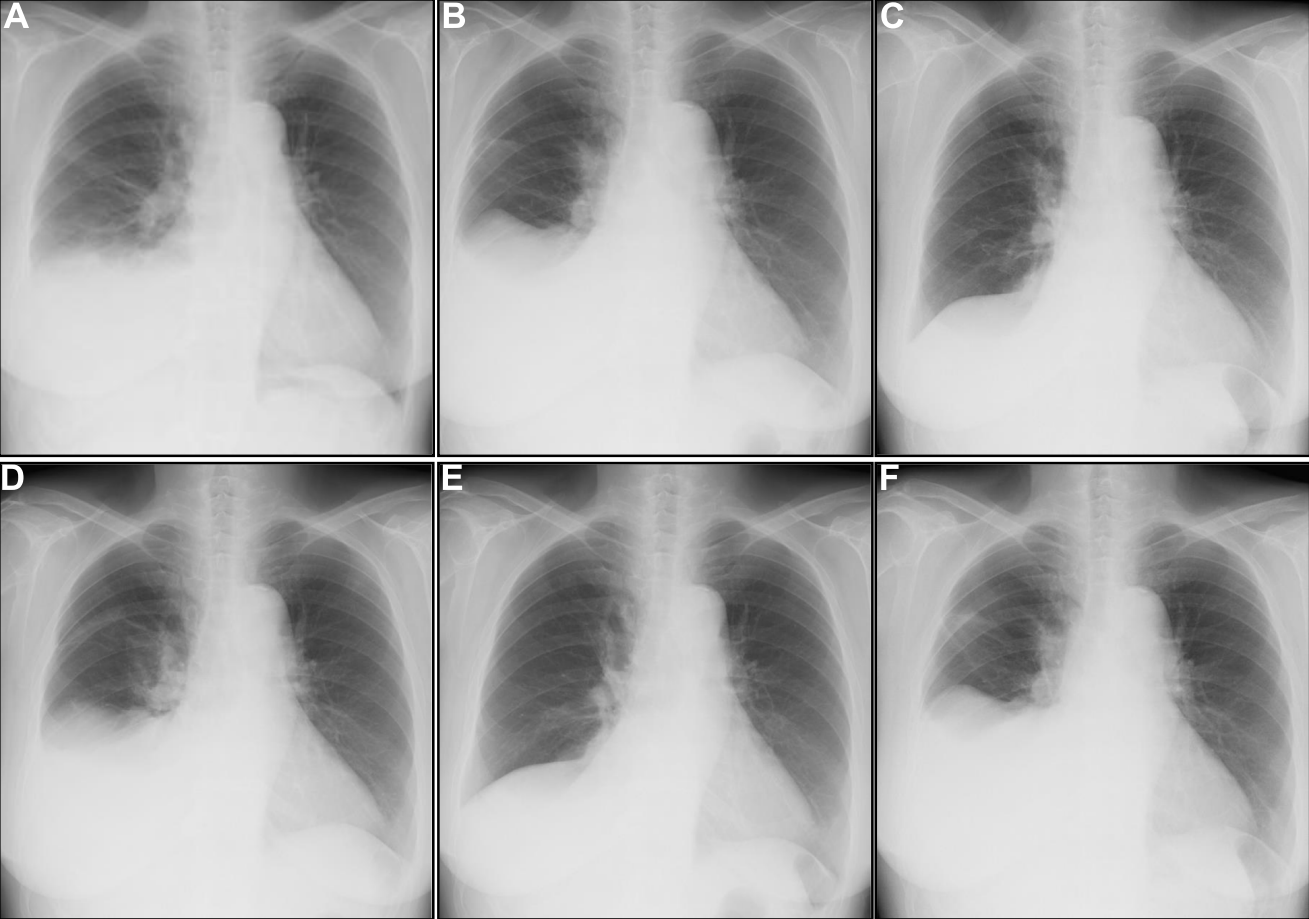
Serial chest radiographs. a On admission; an initial radiograph shows right-sided pleural effusion. b Day 6; radiograph following diuretic therapy. Note, the effusion remains unchanged. c Day 7; radiograph after the removal of 1240 mL effusion via the first thoracentesis shows resolution of effusion. d Day 14; follow-up radiograph after first thoracentesis shows reaccumulation of effusion. e Day 16; radiograph after the removal of 1200 mL effusion via a second thoracentesis shows resolution of effusion. f Day 23; follow-up radiograph following second thoracentesis shows reaccumulation of effusion
